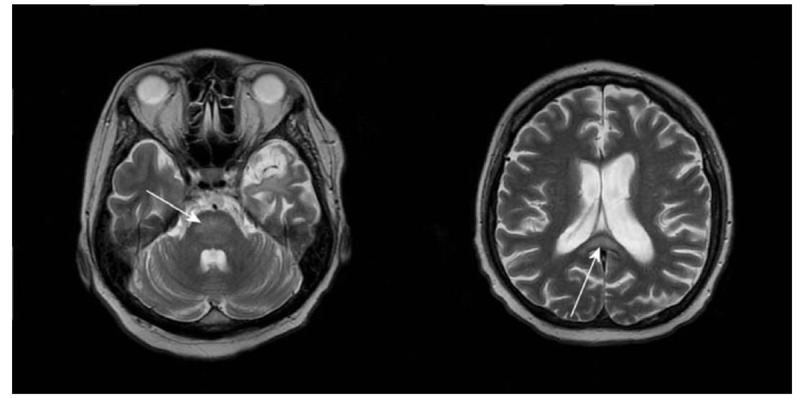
Brain MRI (axial). T2 hyperintensity (white arrow) of the central pons (left) and the splenium of the corpus callosum (right). MRI = magnetic resonance imaging.
